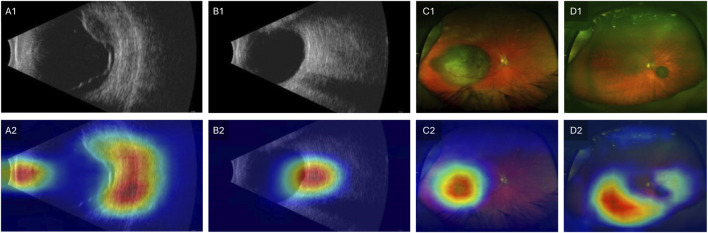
Grad-CAM showing regions relevant for classification. Row 1 shows original images and Row 2 shows the Grad-CAM heatmaps overlaid on the original images. (A1–A2) Ultrasound image of choroidal melanoma. (B1–B2) Ultrasound image of choroidal nevus. (C1–C2) Ultra-widefield retinal image of choroidal melanoma. (D1–D2) Ultra-widefield retinal image of choroidal nevus. Modified with permission from [57].
